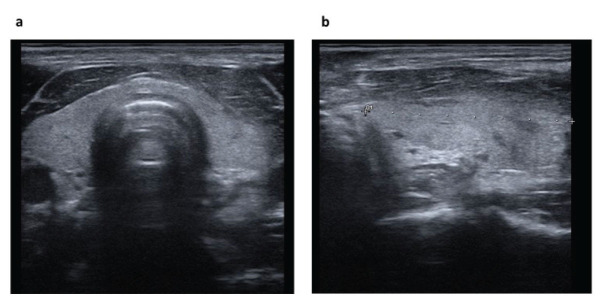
Thyroid ultrasound 3 months after discharge, both in transversal (a) and longitudinal section of the left thyroid lobe (b). A restitutio in integrum of ultrasound thyroid characteristic was observed. In particular, only a slight inhomogeneity in the left thyroid lobe was observed.
